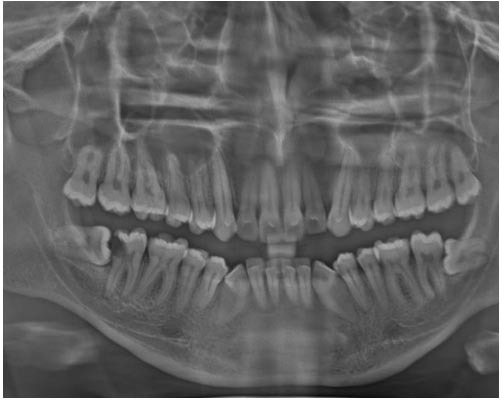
There was no evidence of bone involvement in panoramic radiograph
